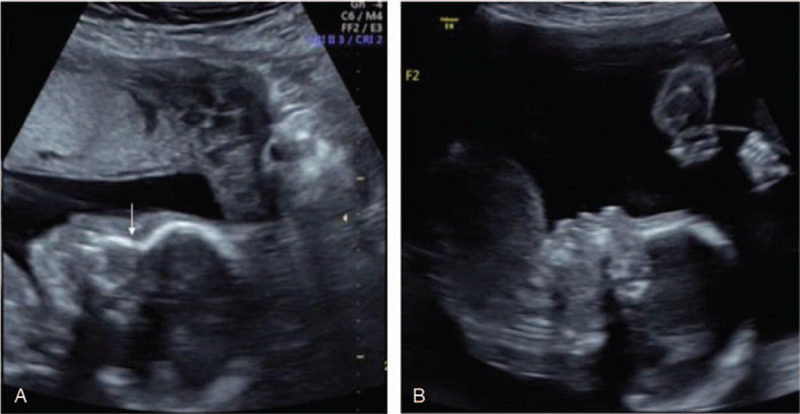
Ultrasound image showing a dichorionic-diamniotic twin pregnancy discordant for the absence of the fetal nasal bone (FNB) at 21 + 2 weeks’ gestation. Sagittal view of the fetal face demonstrating normal nasal bone in twin A (arrow) (A) and an abnormal profile with the absence of the nasal bone in the affected twin B (B).
